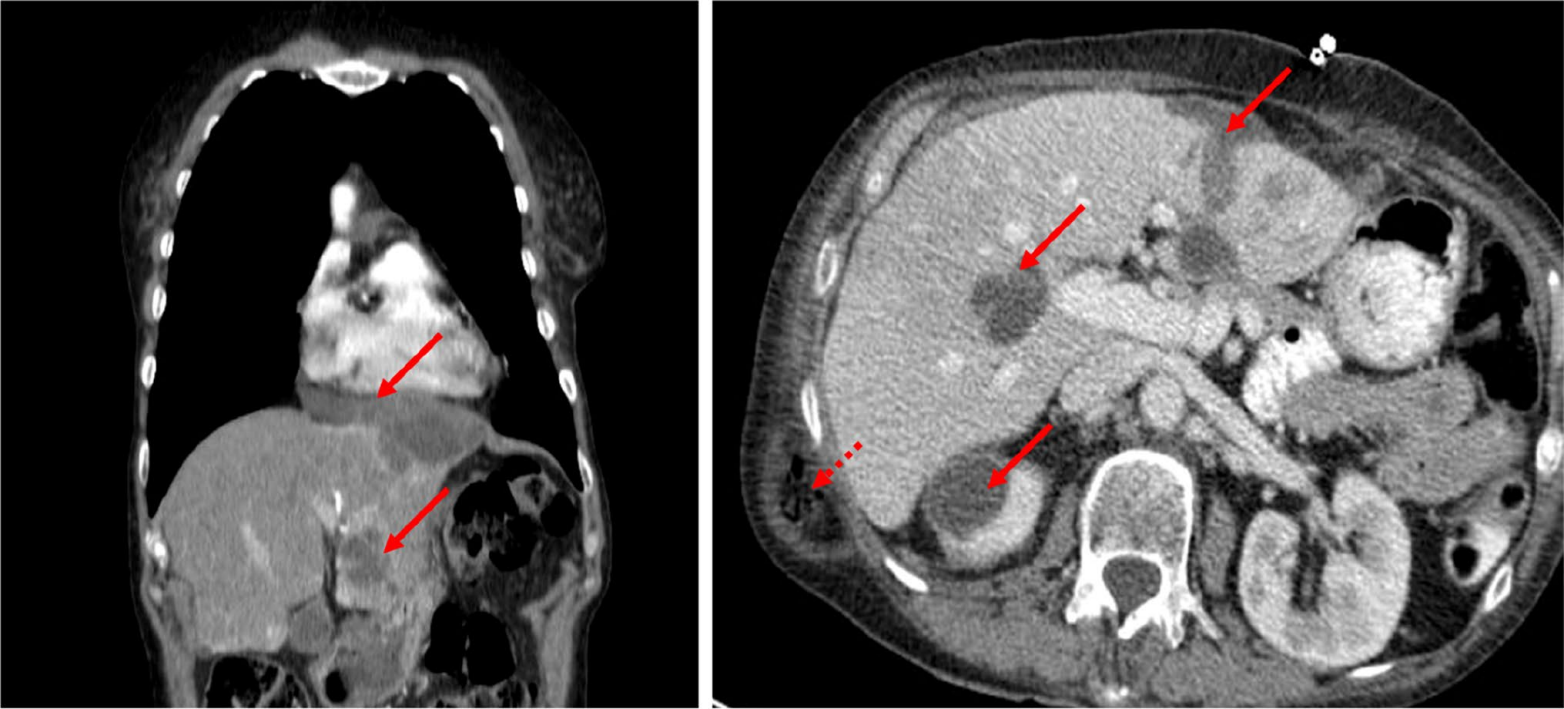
Computed tomography scan after 2 years of treatment with crizotinib showing abscess formations (arrows) in the liver, the thoracic wall and kidney. The dashed arrow indicates a subcutaneous hernia
